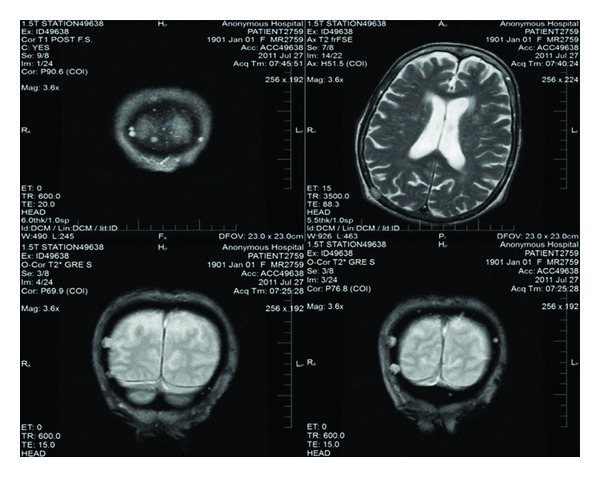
MRI demonstrating lytic lesions.
